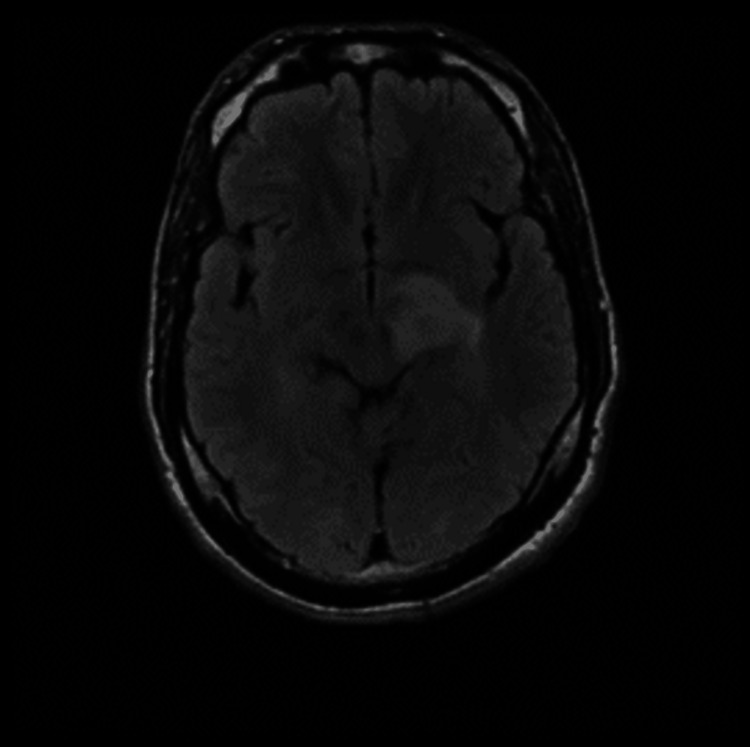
Brain MRI revealed abnormal signal intensity lesion seen at the left thalamus, posterior limb of the internal capsule, and left superior cerebellar peduncle reflecting hyperintense signals at FLAIR WI. FLAIR WI: fluid-attenuated inversion recovery-weighted image
